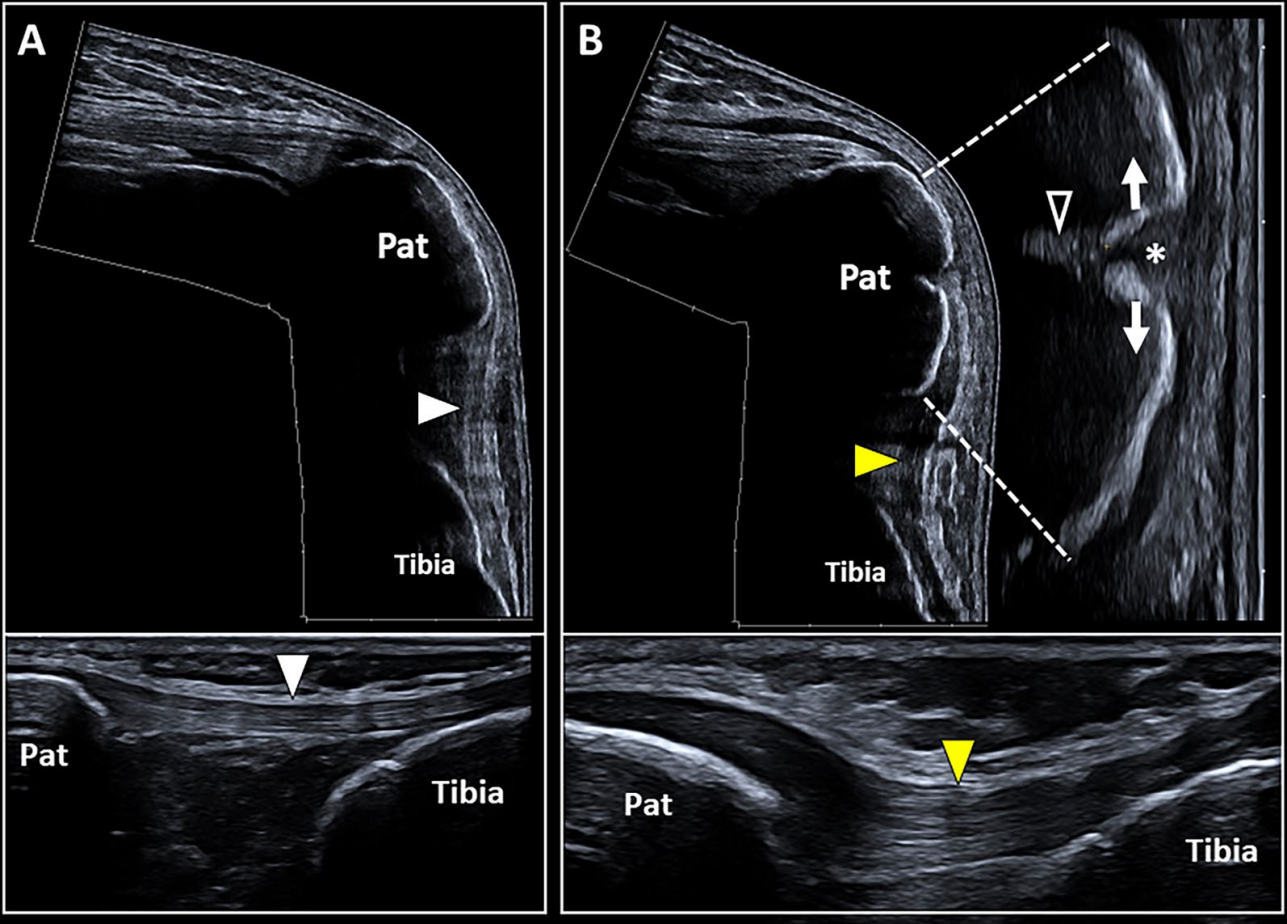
Pathological sonographic findings of the bone tissue. The comparative sonographic assessment shows a continued hyperechoic cortical bone of the patella (*Pat*) with a tensioned patellar ligament (*white arrowhead*) on the healthy side (A); instead, cortical defect (*white asterisk*) of the patella (*Pat*), diastasis of bony fragments (*white arrows*), and deformation of the patellar ligament (*yellow arrowhead*) are clearly visible in the post-traumatic knee (B). Of note, the disruption of the bony cortex allows the US beam to partially penetrate within the bone tissue generating an echoic wedge (*void arrowhead*) (B)
